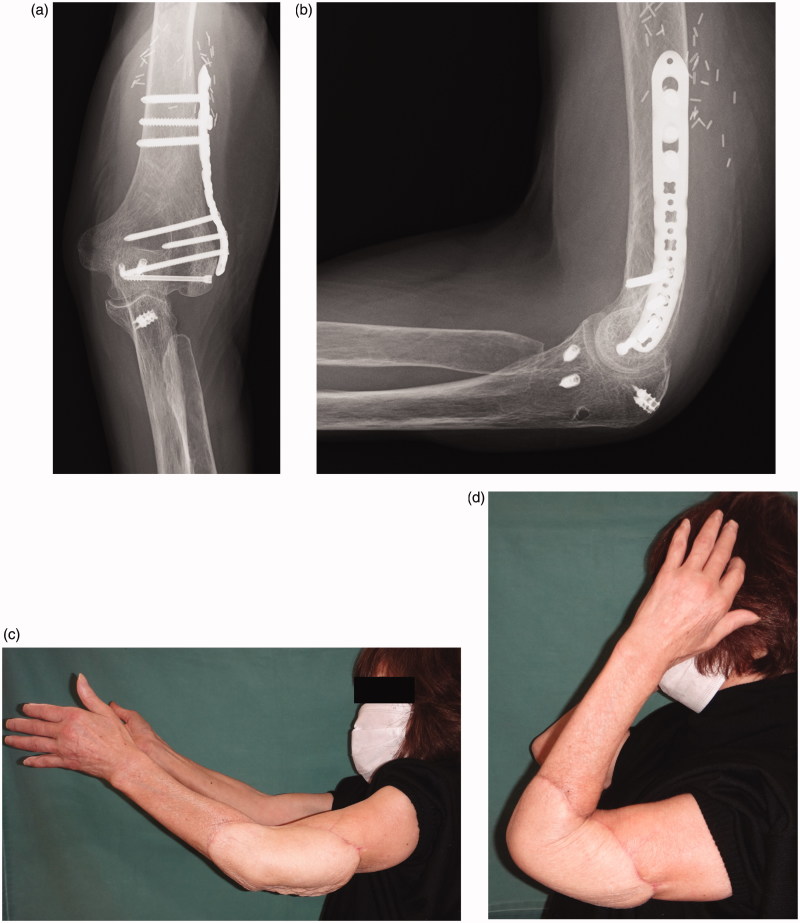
Follow-up radiograph and photograph obtained 12 months after surgery. Anterior-posterior (a) and lateral (b) radiographs showing bony union of the osteotomy site, with no evidence of osteoarthritic changes at the elbow joint. Clinical photograph showing functional results in extension (c) and flexion (d).
